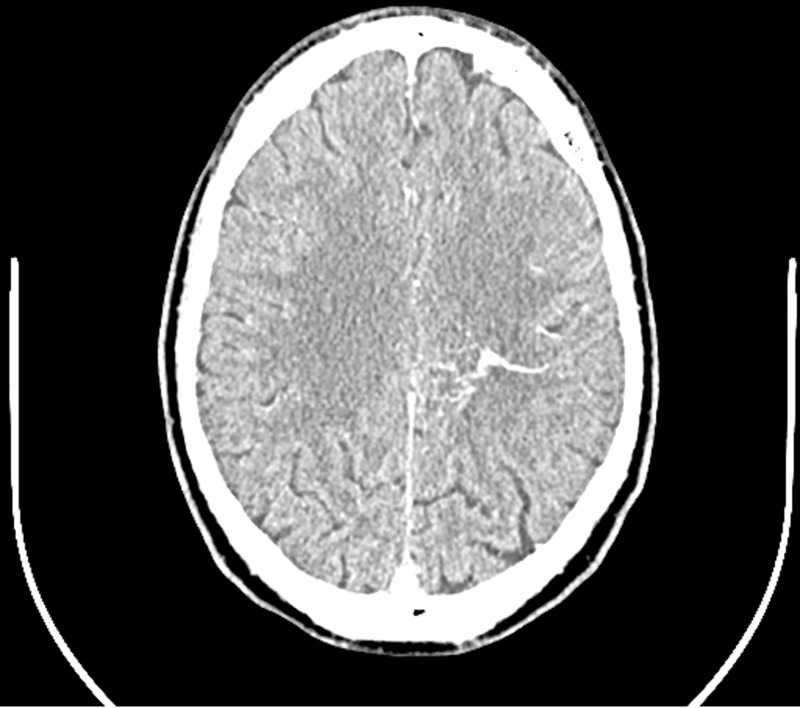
post-contrast axial computed tomography brain demonstrating the characteristic “caput medusae” sign of a developmental venous anomaly, formed by multiple small medullary veins converging into a larger collector vein
